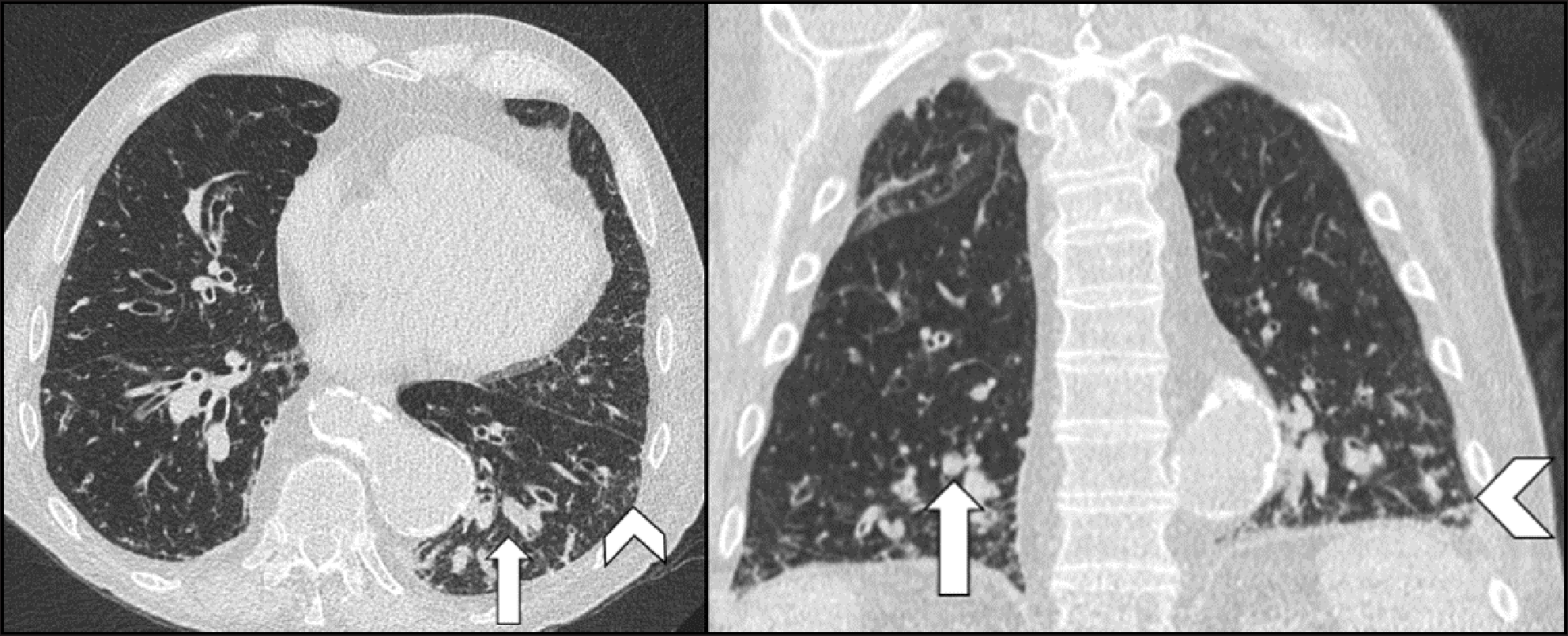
Low-dose chest CT of a patient with known chronic obstructive pulmonary disease with findings inconsistent (negative) for COVID-19 pneumonia. No typical findings for COVID-19 were seen. Bronchiectasis with mucus plugs (arrows) and discrete zones of tree-in-bud (arrowheads) are shown. RT-PCR was negative for this patient. Patient was treated for acute exacerbation.
